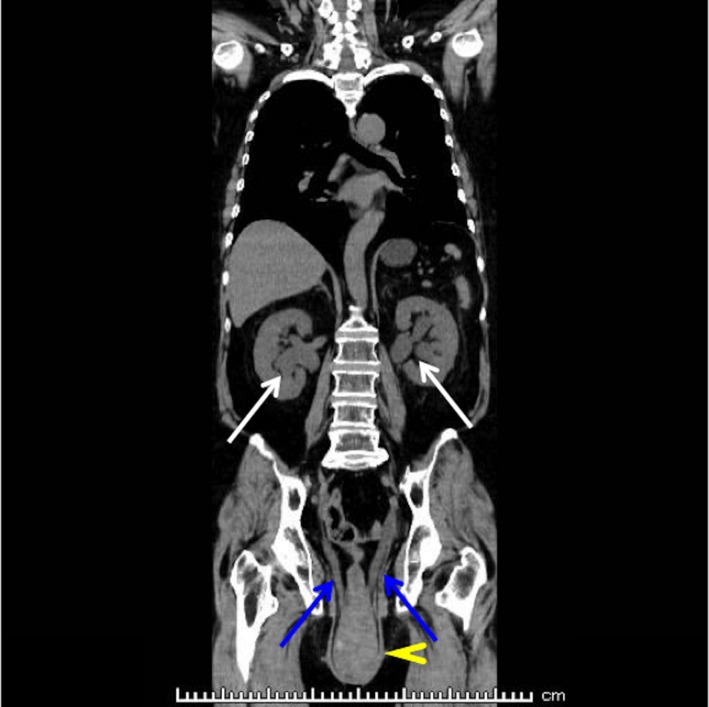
Computed tomography (CT) imaging findings. Coronal view shows total uterine prolapse (yellow arrowhead) with bilateral hydronephrosis (white arrow) and stretched hydroureter (blue arrow)
